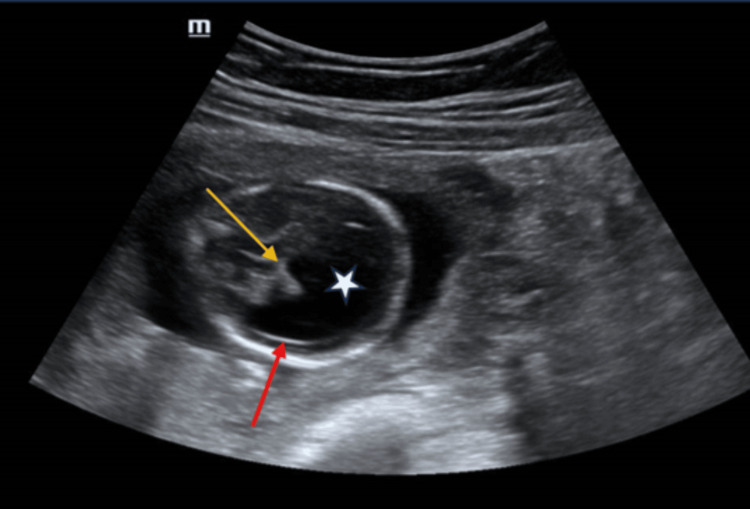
Axial ultrasound image of the fetal brain shows a large central monoventricle (white star) with a surrounding small rim of cerebral cortex (red arrow) and fused thalami (yellow arrow).
